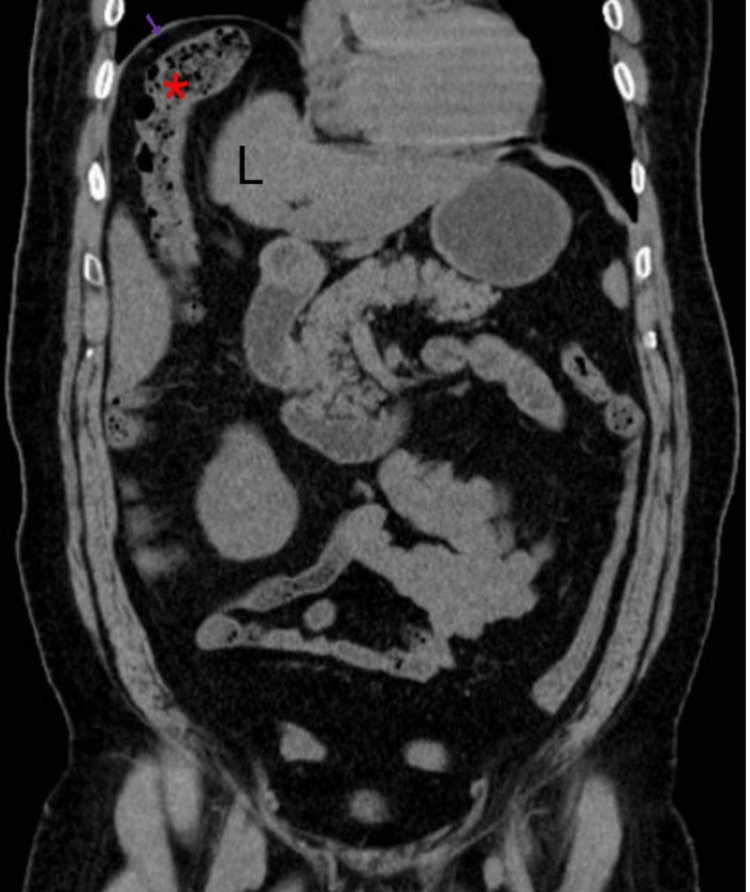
Coronal non-contrast computed tomography (CT) showing interposition of the large bowel (*) in between the liver (L) and diaphragm (shown by arrow) in accordance with Chilaiditi syndrome
